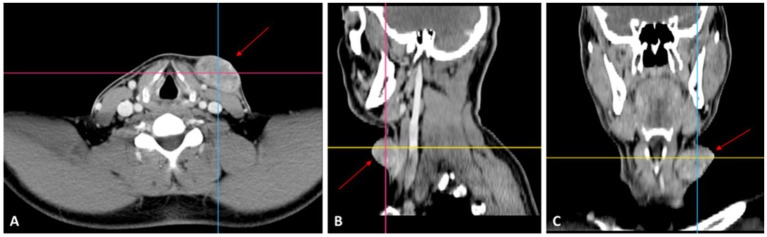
Contrast-enhanced CT evaluation of the lesion. (A) Axial image showing a well-defined subcutaneous mass (red arrow). (B) Sagittal image demonstrating confinement to the subcutaneous plane (red arrow). (C) Coronal image showing displacement without invasion of adjacent structures (red arrow).
